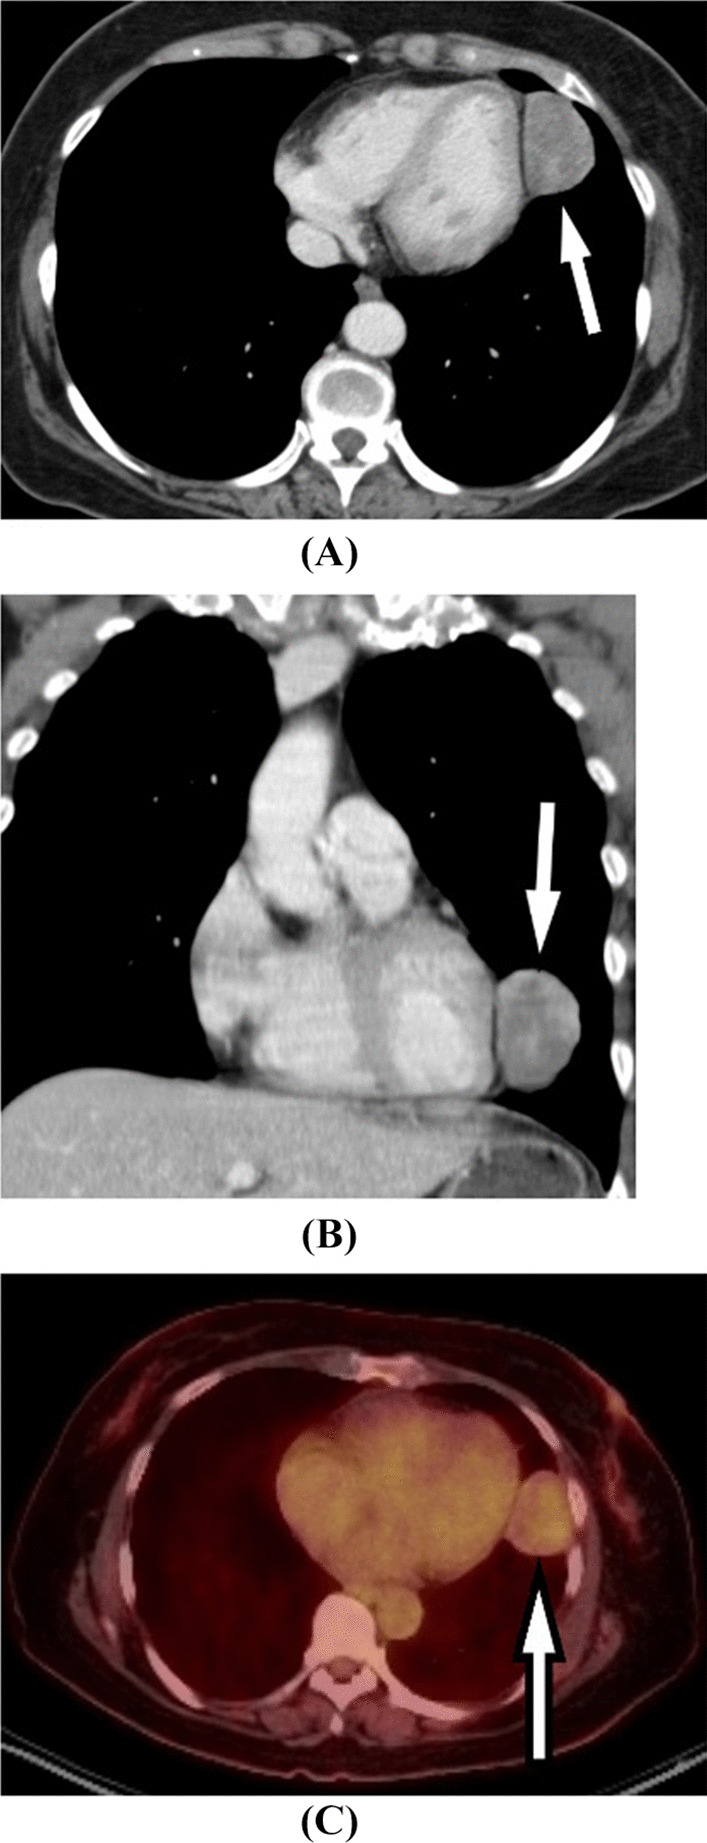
A Axial and B coronal CT image of the chest with IV contrast demonstrates a heterogenous mass (arrow) forming obtuse angles with the mediastinal pleura. C Axial PET-CT image demonstrates the mass (arrow) with moderate FDG uptake
